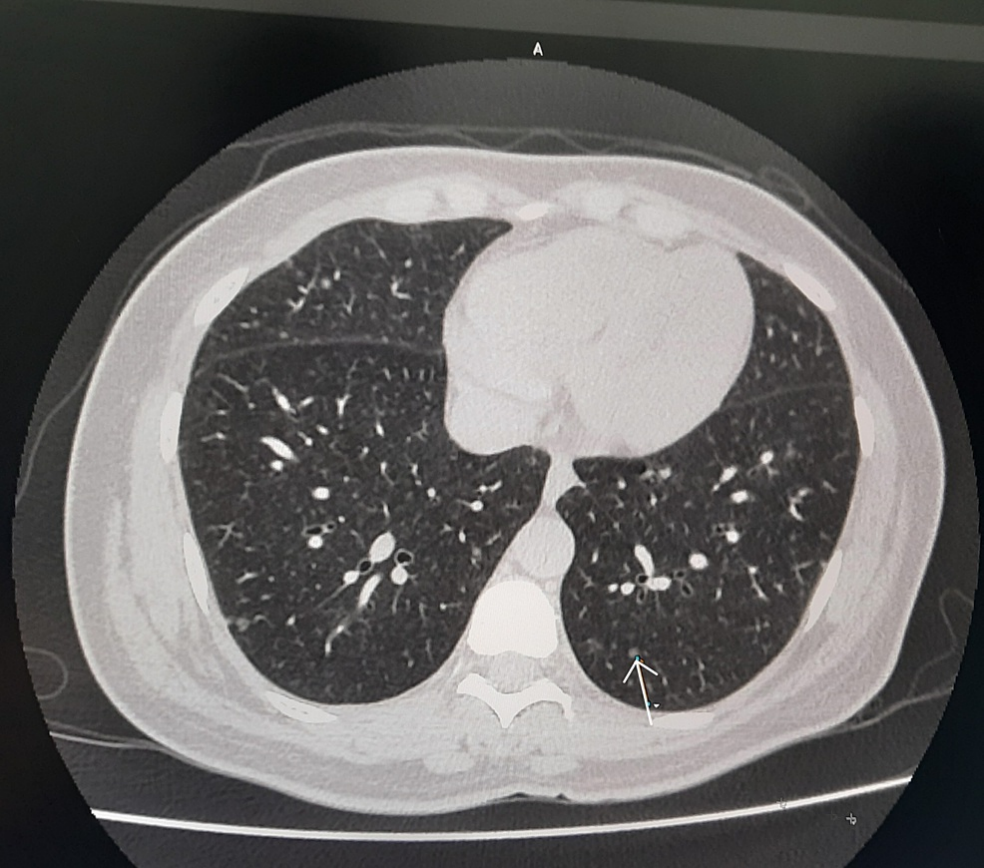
High-resolution computed tomography (HRCT) chest showing multiple 3-5 mm-size pulmonary nodules seen in all lobes of both lungs favor multifocal micronodular pneumocyte hyperplasia (MMPH) with no features of lymphomyomatosis
